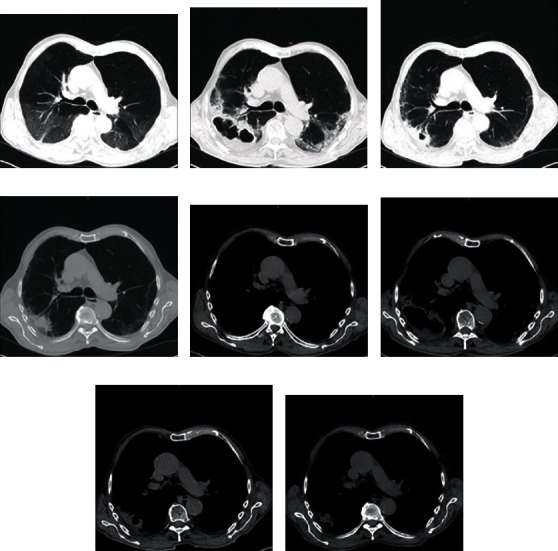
Axial chest CT scans showing peripheral ground-glass opacities of both lungs at hospital admission (a, e), a large cavitary lesion at the right inferior lung lobe at the end of corticosteroid treatment (b, f), a declining cavitary lesion at the end of antibacterial therapy (c, g), and a small consolidation at two-month follow-up (d, f).
